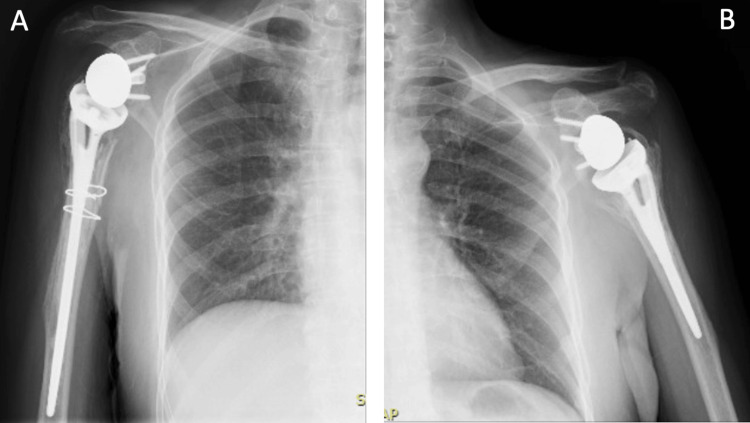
X-ray anteroposterior view of the right (A) and left (B) shoulders and humeri (5 months post-operative) shows no signs of loosening or scapular notching.
